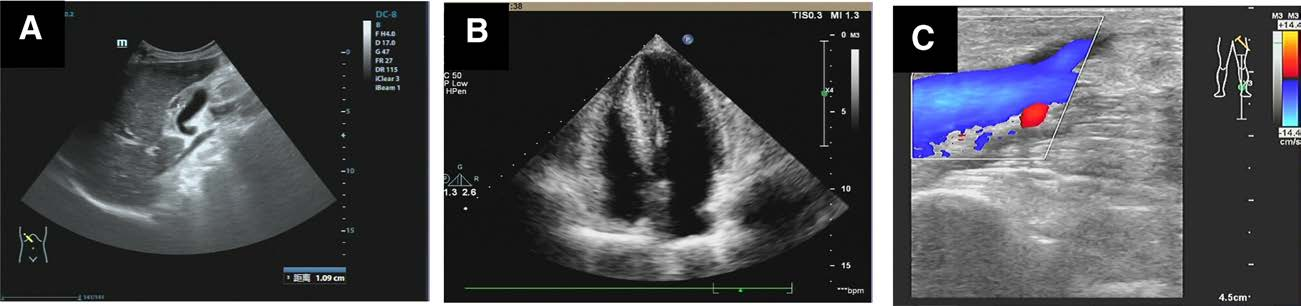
Ultrasonography. (A) Abdominal ultrasonography (October 8, 2021): the oblique diameter of the right lobe of the liver is 158 mm; the anterior–posterior diameter of the left lobe is 112 mm; and the longitudinal diameter of the left lobe is 104 mm. The capsule is smooth. The photoelectric distribution in the liver is uniform, and the distribution of liver blood vessels is clear. The inner diameter of the main portal vein is about 10 mm. The left kidney is not detected (excised), and the right kidney is approximately 125 × 46 mm, with a clear contour and slightly enhanced parenchymal echo. No separation is observed in the right renal collecting system, and CDFI showed abundant blood supply to the right kidney. (B) Cardiac ultrasonography (November 29, 2021): The diameter of each chamber is within the normal range. The left ventricular wall is thickened, and there are no obvious abnormalities in the morphology and structure of each valve. The thickness of the fluid (dark area) in the anterior pericardial cavity is about 3 mm, and the thickness of the fluid (dark area) in the right ventricular pericardial cavity is about 4 mm. Doppler ultrasonography showed mild mitral regurgitation, a forward flow velocity of approximately 180 cm/s in the left ventricular outflow tract, and a peak pressure difference of approximately 13 mm Hg. Mitral valve spectral Doppler shows an E/A ratio < 1. Left ventricular systolic function was as follows: ejection fraction, 74%; fractional shortening, 43%, presenting with congestive heart failure. (C) Bilateral lower limb venous ultrasonography shows no significant abnormalities (February 6, 2022).
